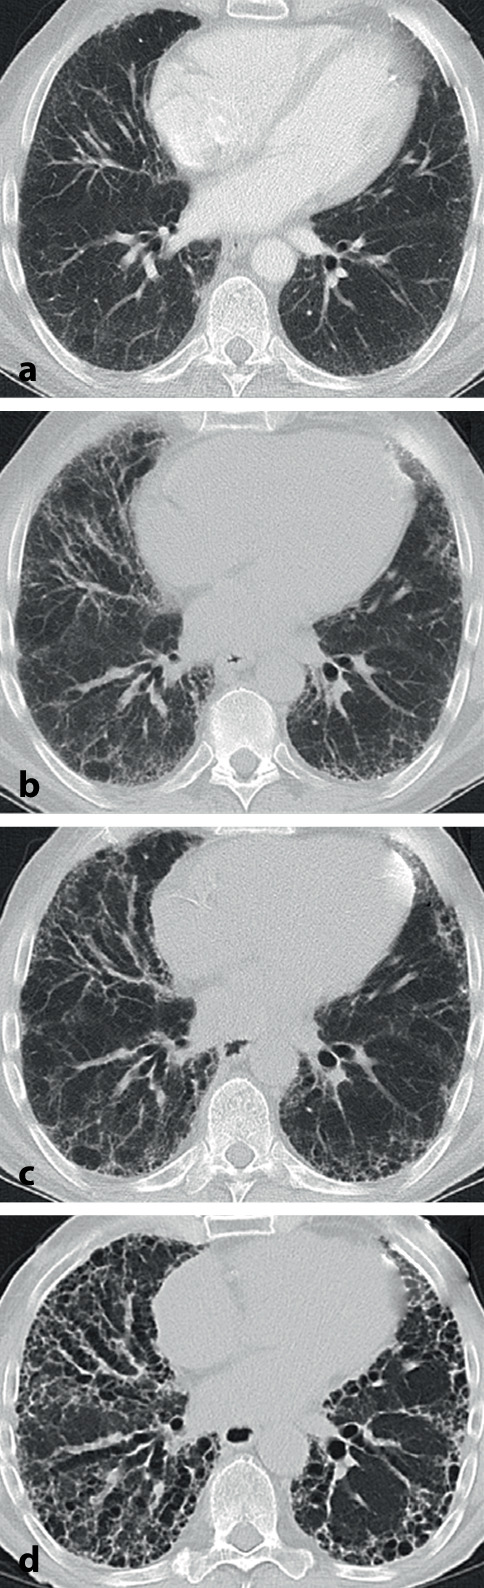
Neben der Unterscheidung verschiedener Muster ermöglicht die HRCT in gewissem Maße auch eine Abschätzung der Prognose [27]. Das klassische UIP-Muster mit Honeycombing und Traktionsbronchiektasen hat – ungeachtet seiner Ursache – die ungünstigste Prognose [1, 3, 27, 30]. Muster mit Milchglasverdichtungen oder retikulären Verdichtungen, wie z. B. das der NSIP, sind prognostisch günstiger und oftmals einer immunsuppressiven Therapie eher zugänglich (Abb. 3; [31, 32]) Dennoch können auch primär überwiegend inflammatorische Veränderungen später zu irreversibler Fibrose führen bzw. liegen oft von Beginn an Mischbilder aus fibrotischen und inflammatorischen Veränderungen vor.
